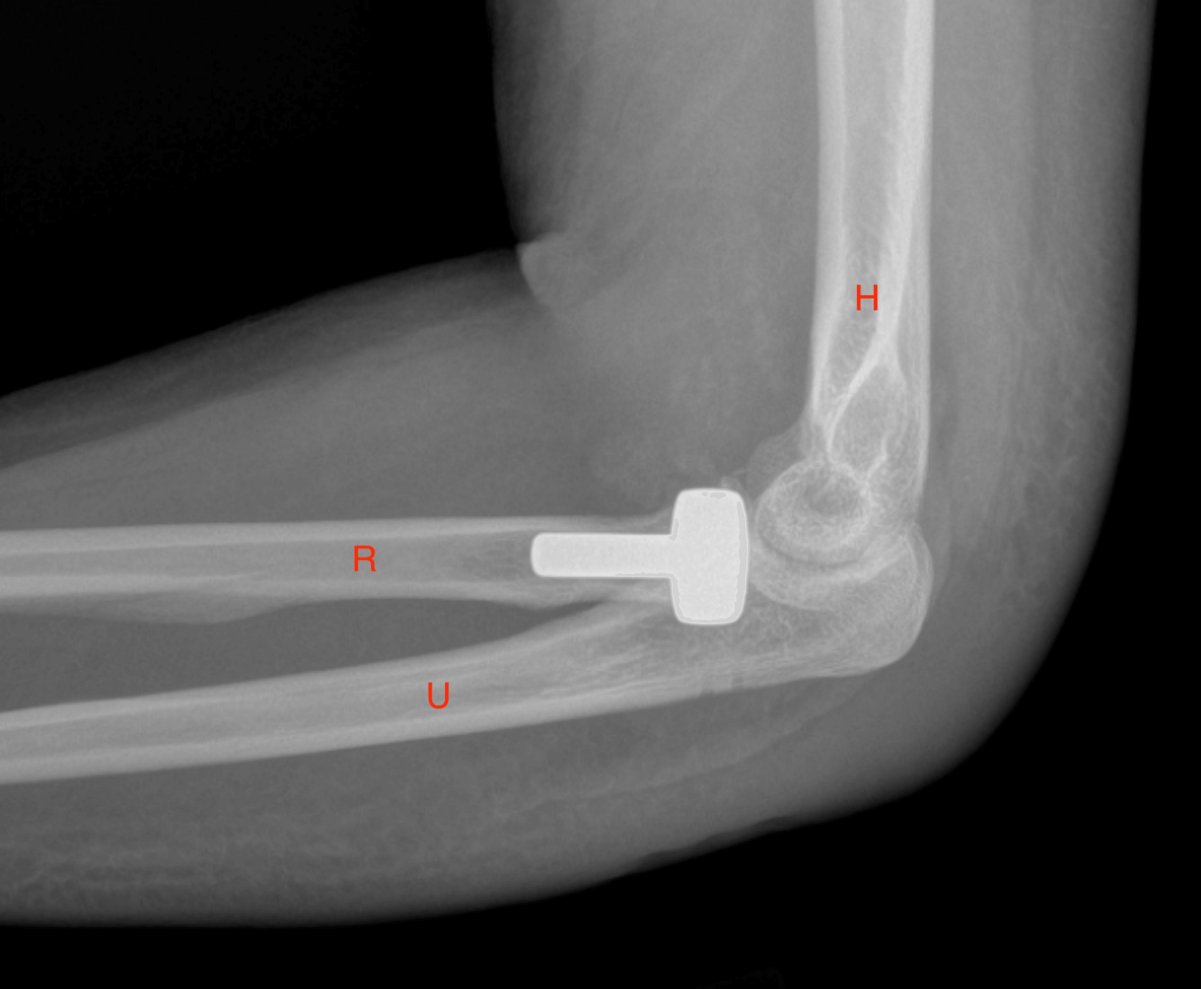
An X-ray of the left elbow after surgery with radial head replacement intact. H: humerus; R: radius; U: ulna
